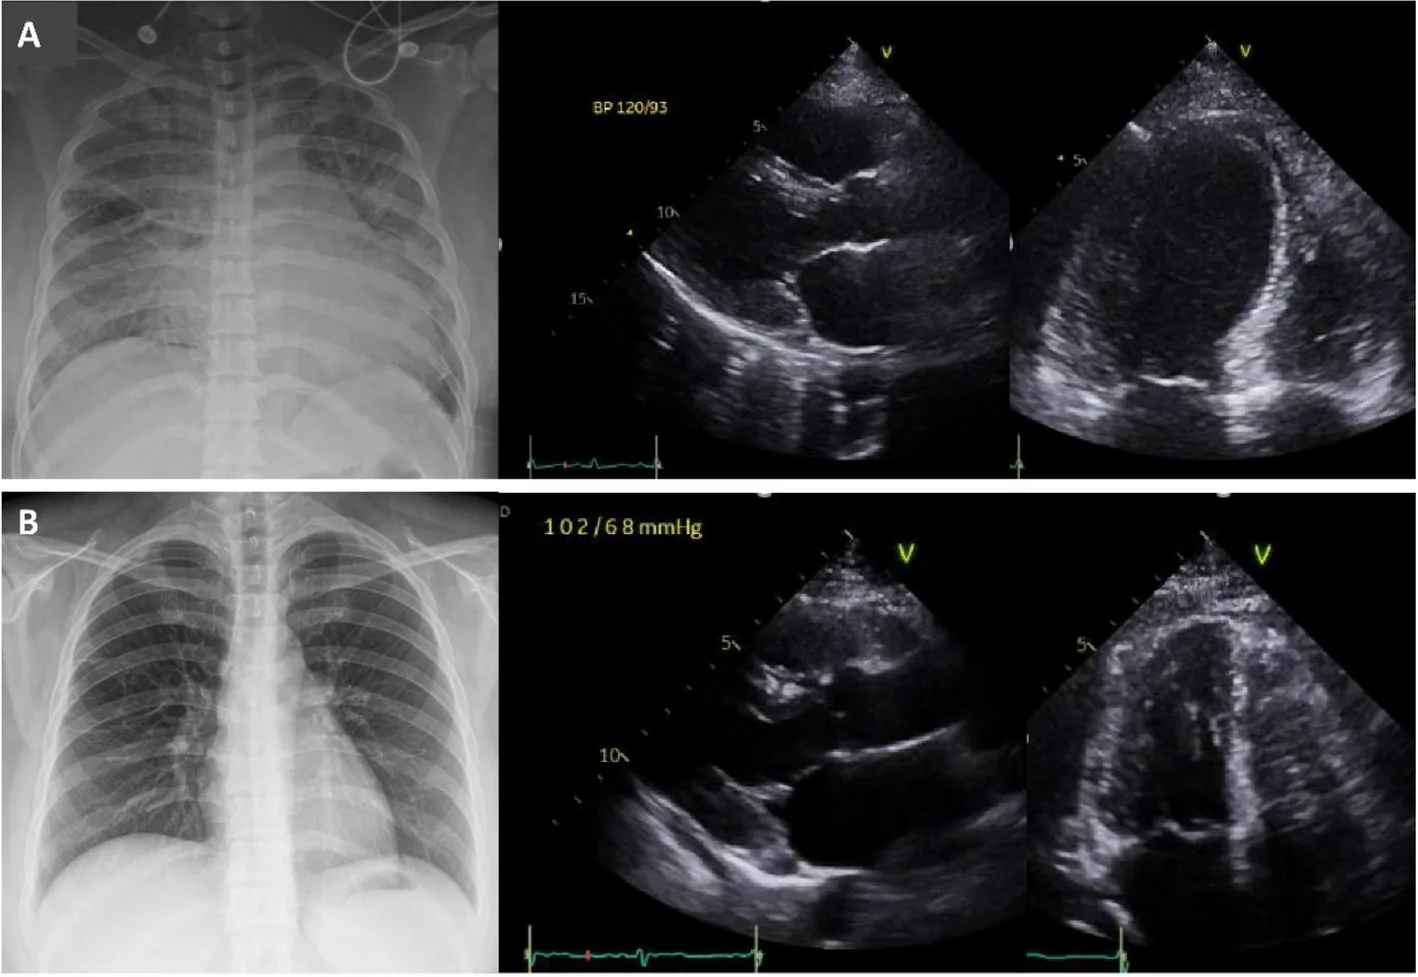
Peripartum cardiomyopathy. A A 39-year-old woman with peripartum cardiomyopathy presented with dyspnea immediately after delivery of twins. Chest x-ray demonstrated pulmonary edema, and echocardiography revealed severe left ventricular dysfunction. B At 2-month follow-up, left ventricular function had completely recovered. All echocardiographic images are in end-systole
